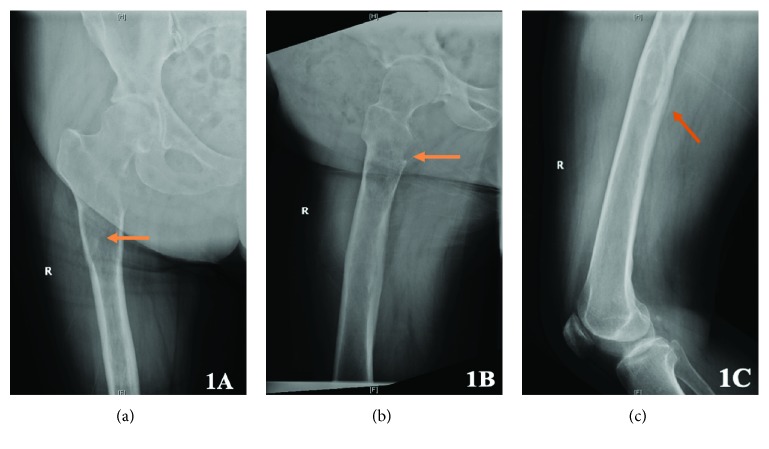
Initial anteroposterior and lateral radiographs of the right femur. (a) An expansile intramedullary lucent lesion is seen within the subtrochanteric region with no evidence of chondroid calcification. (b) Cortical destruction and lytic expansion of the lesser trochanter. (c) Ground-glass appearance of the mid femoral shaft with a sclerotic rim compatible with fibrous dysplasia.
